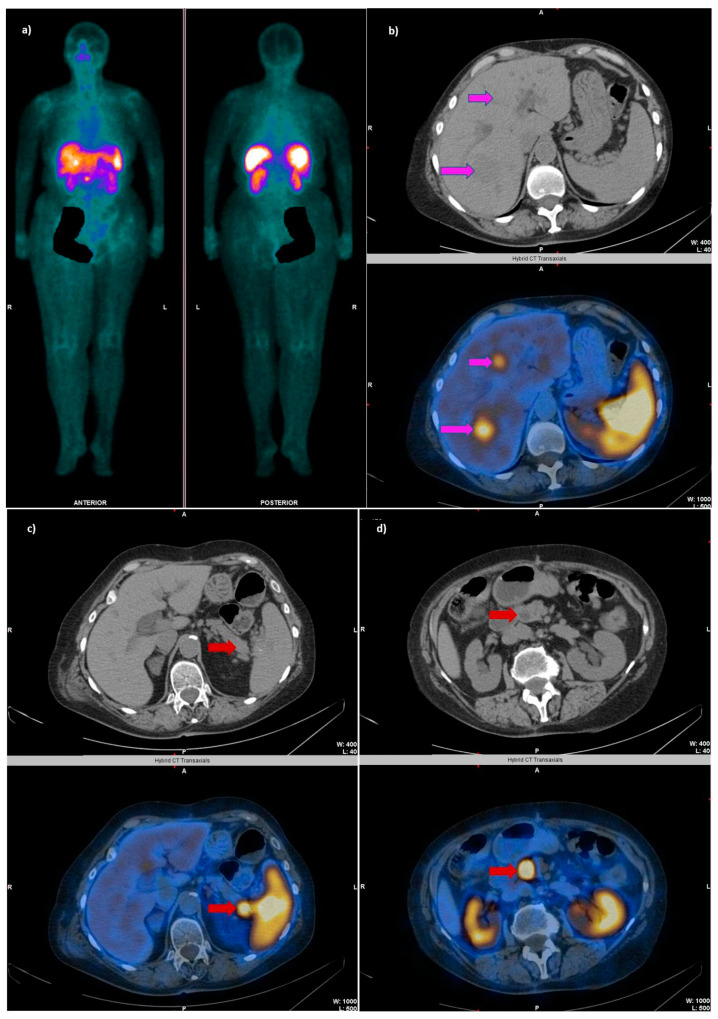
A 69-year-old female patient diagnosed with a pancreatic neuroendocrine tumor (confirmed through multiple biopsies from the pancreatic head and liver metastasis as NET G2), undergoing treatment with somatostatin analogs. The WB SRS revealed ^99m^Tc-EDDA/HYNIC-TOC uptake in the abdominal region (a), with the SPECT/CT showing SSTR-expressing liver metastases (b) and two foci of increased uptake in the pancreas: one corresponding to the tail of the pancreas (c) and one in the head of the pancreas (d).
